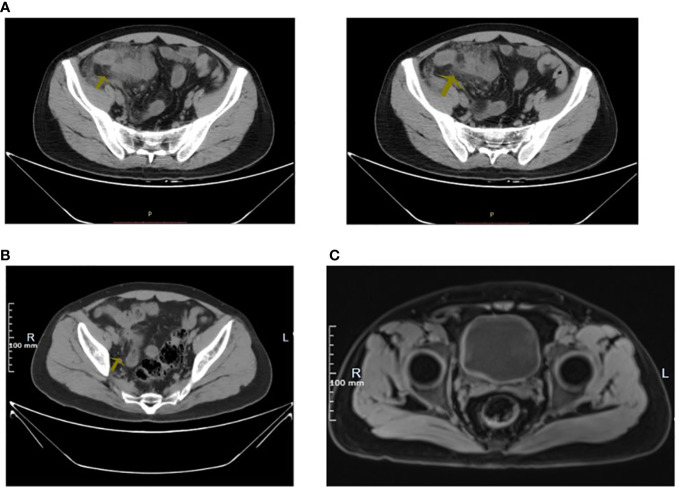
(A) Hypogastrium CT in July 2021: The arrow displayed that there was unclear appendix, the cloudy surrounding fat space, and a little free gas. (B) Hypogastrium CT in November 2022: The arrow displayed that there was slightly entangled intestine with the blurred surrounding fat space in the right pelvic cavity. (C) MRI: The arrow displayed that the bladder filled well.
